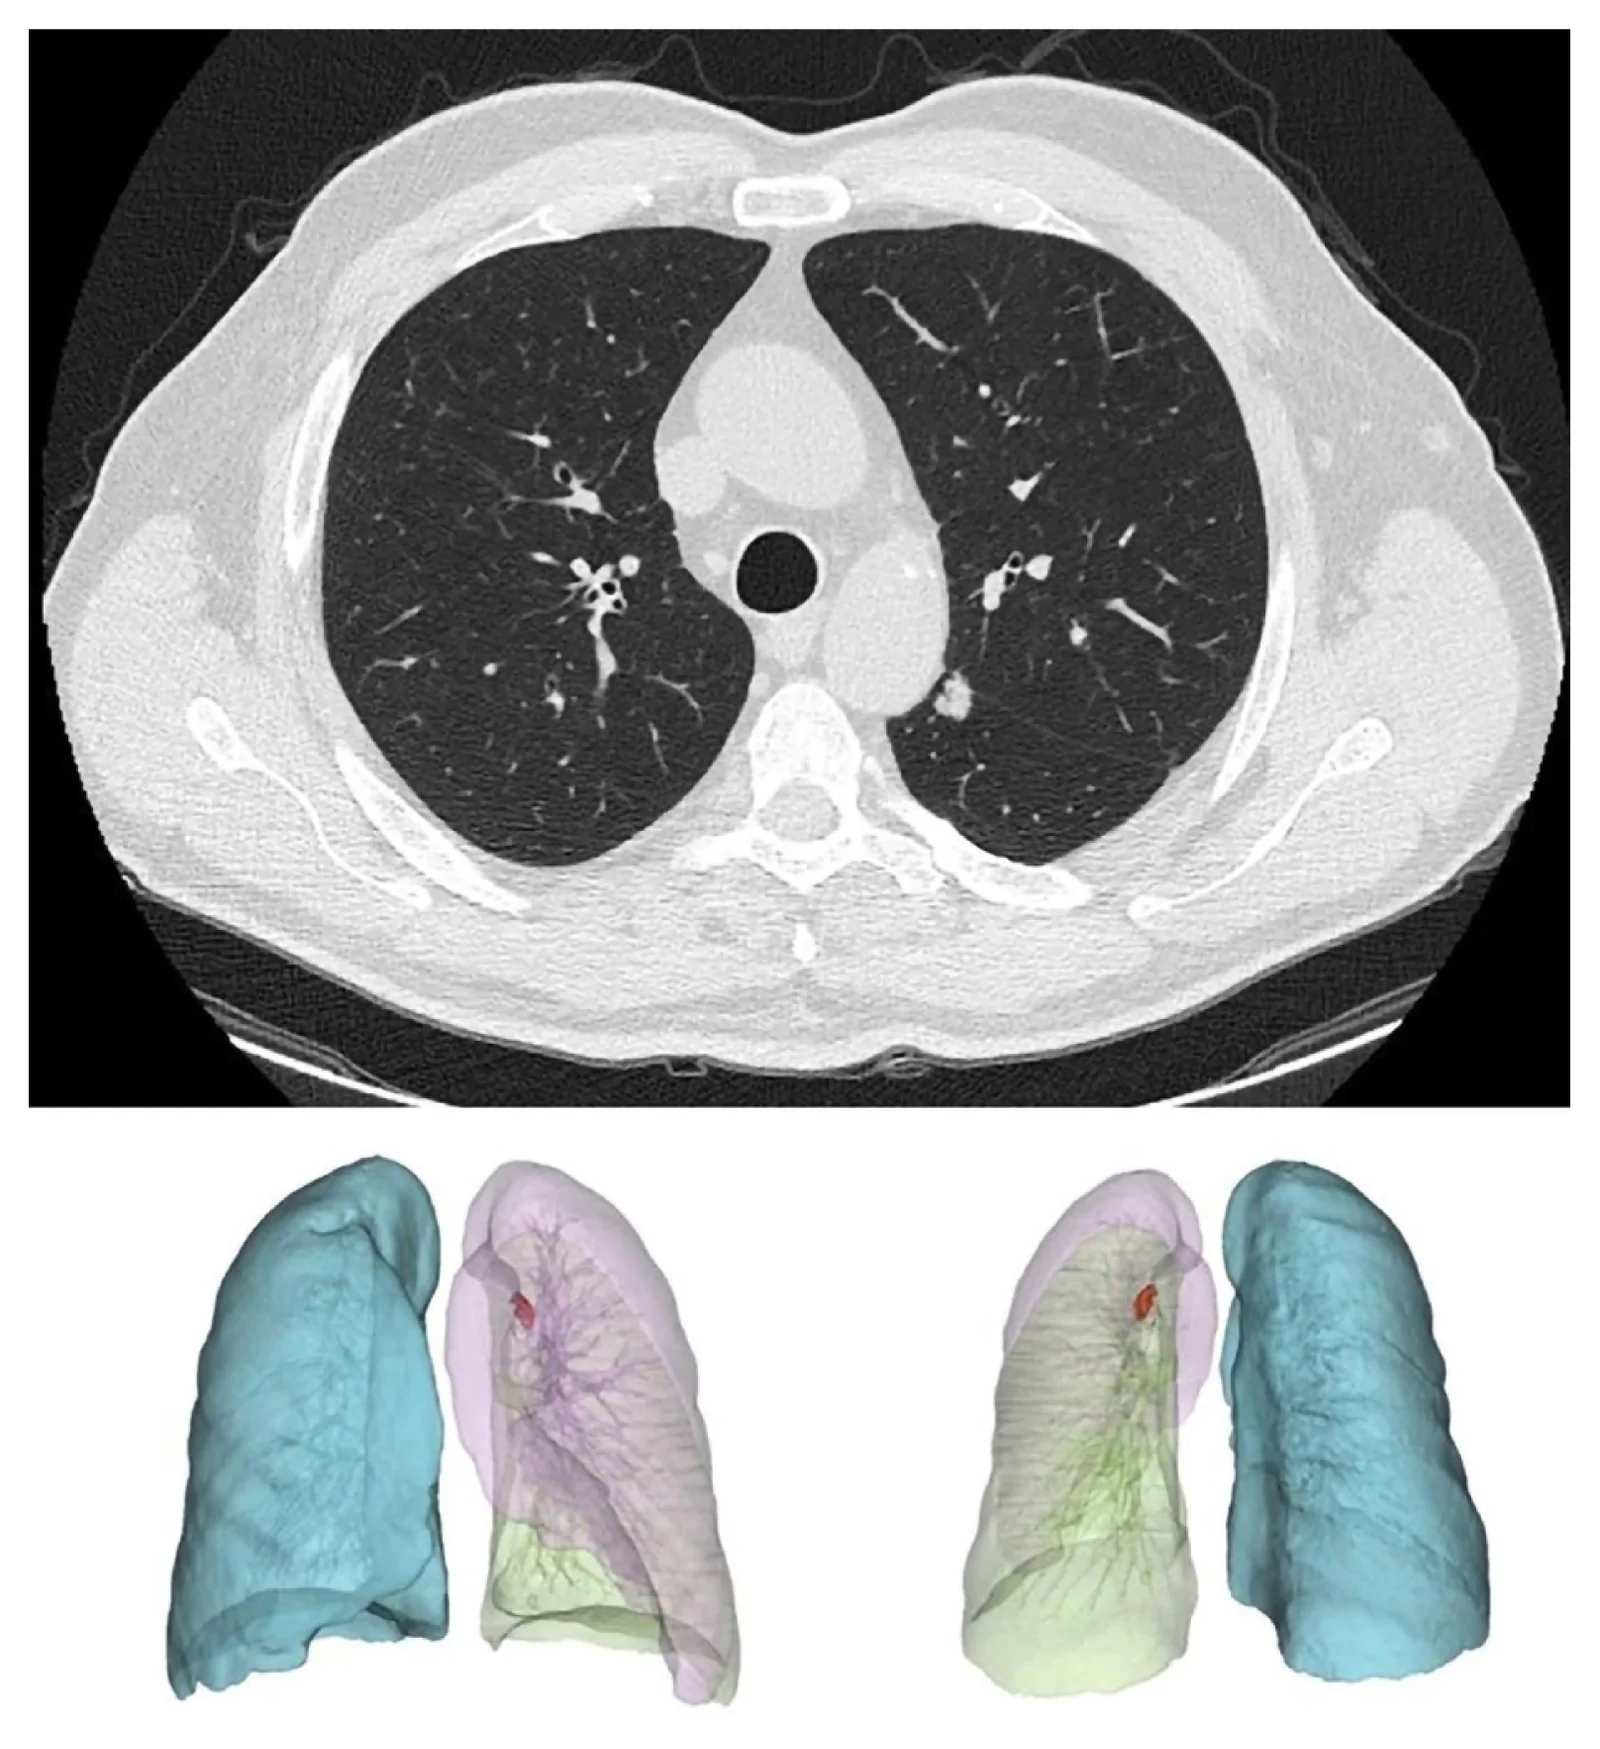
Axial section and 3D reconstruction of the CT of a patient included in the study.
